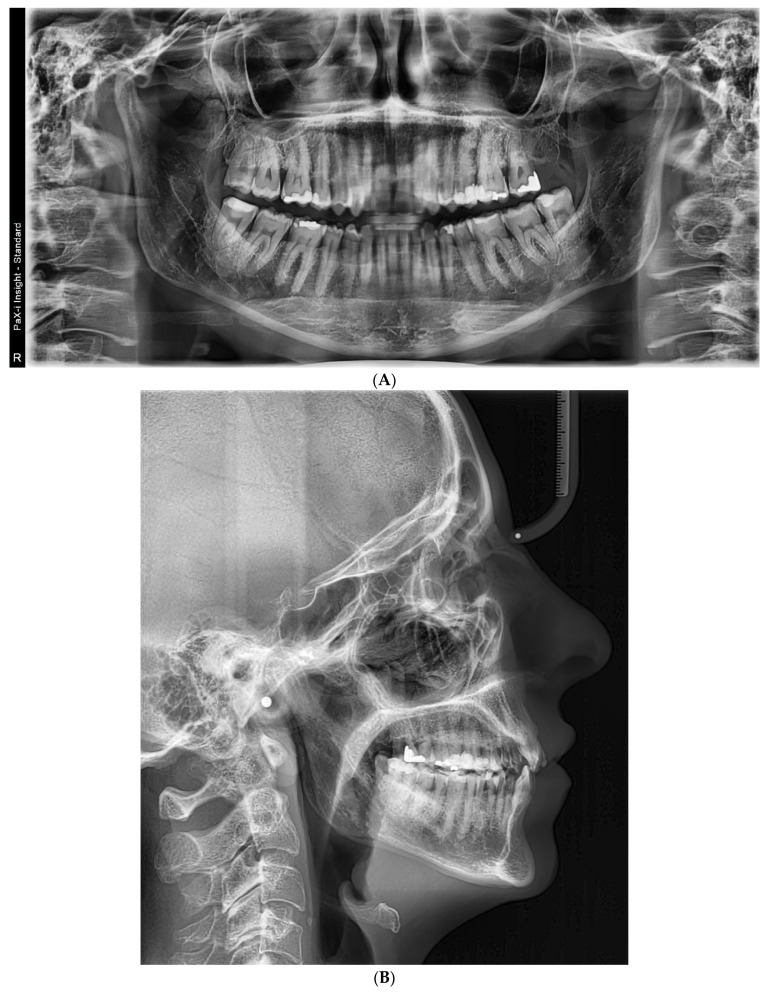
(A) Initial panoramic radiograph; (B) lateral cephalogram.
